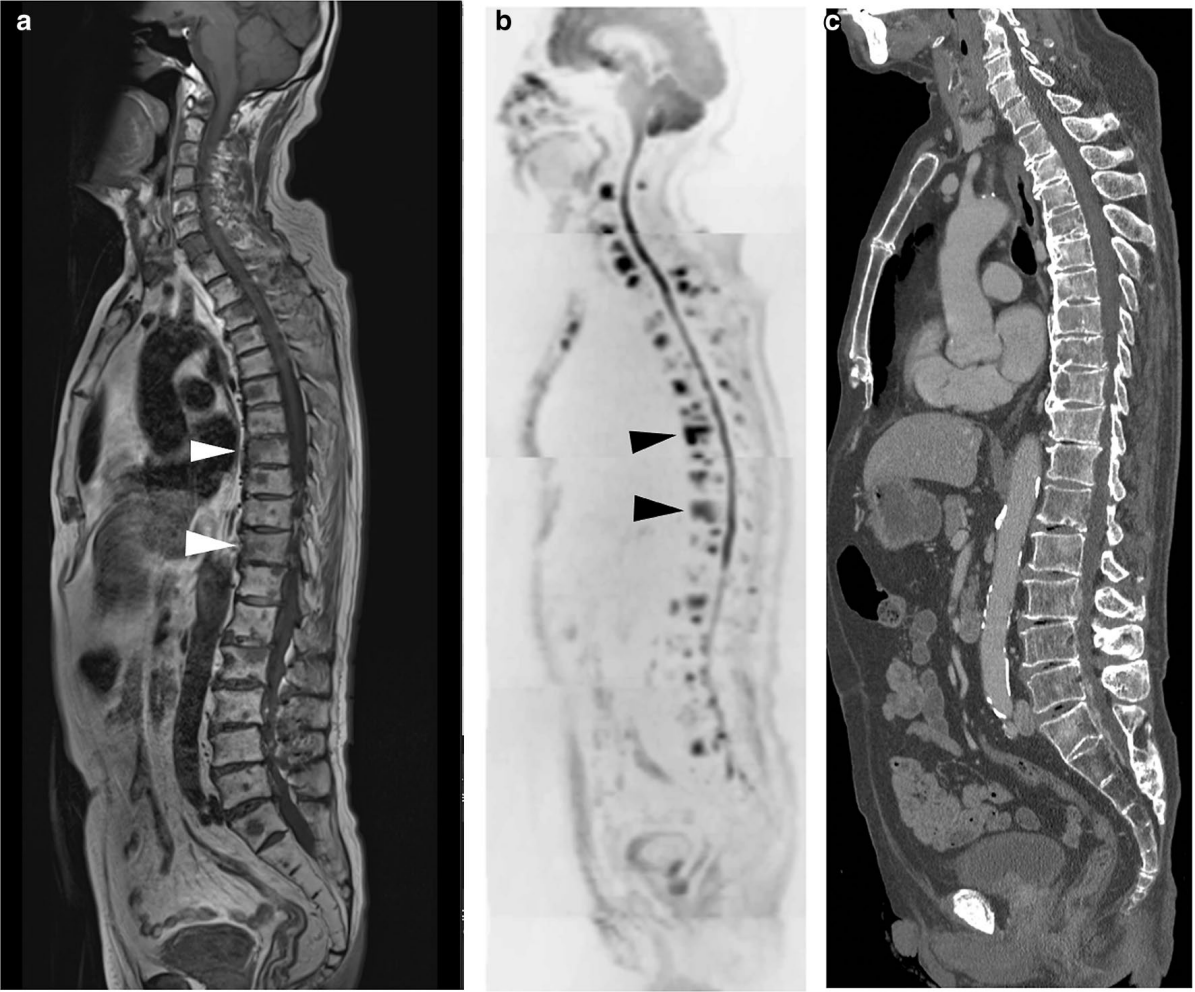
Mixed-type/intertrabecular metastases. A 78-year-old man with a 4-year history of prostate cancer and transition to castration-resistant prostate cancer (CRPC). Serum PSA was 5.954 ng/mL. a On T1W image, multiple low-intensity areas are shown, including in the Th8 and Th11 (white arrowheads). b On the DW sagittal reconstructed image, multiple high-intensity areas are shown, including in the Th8 and Th11(arrowheads). c On the CT reconstructed sagittal image, obvious sclerotic or lytic changes were not observed. In fact, the diagnosis of multiple bone metastases using only CT examination was not possible. These CT findings were defined as intertrabecular metastases
